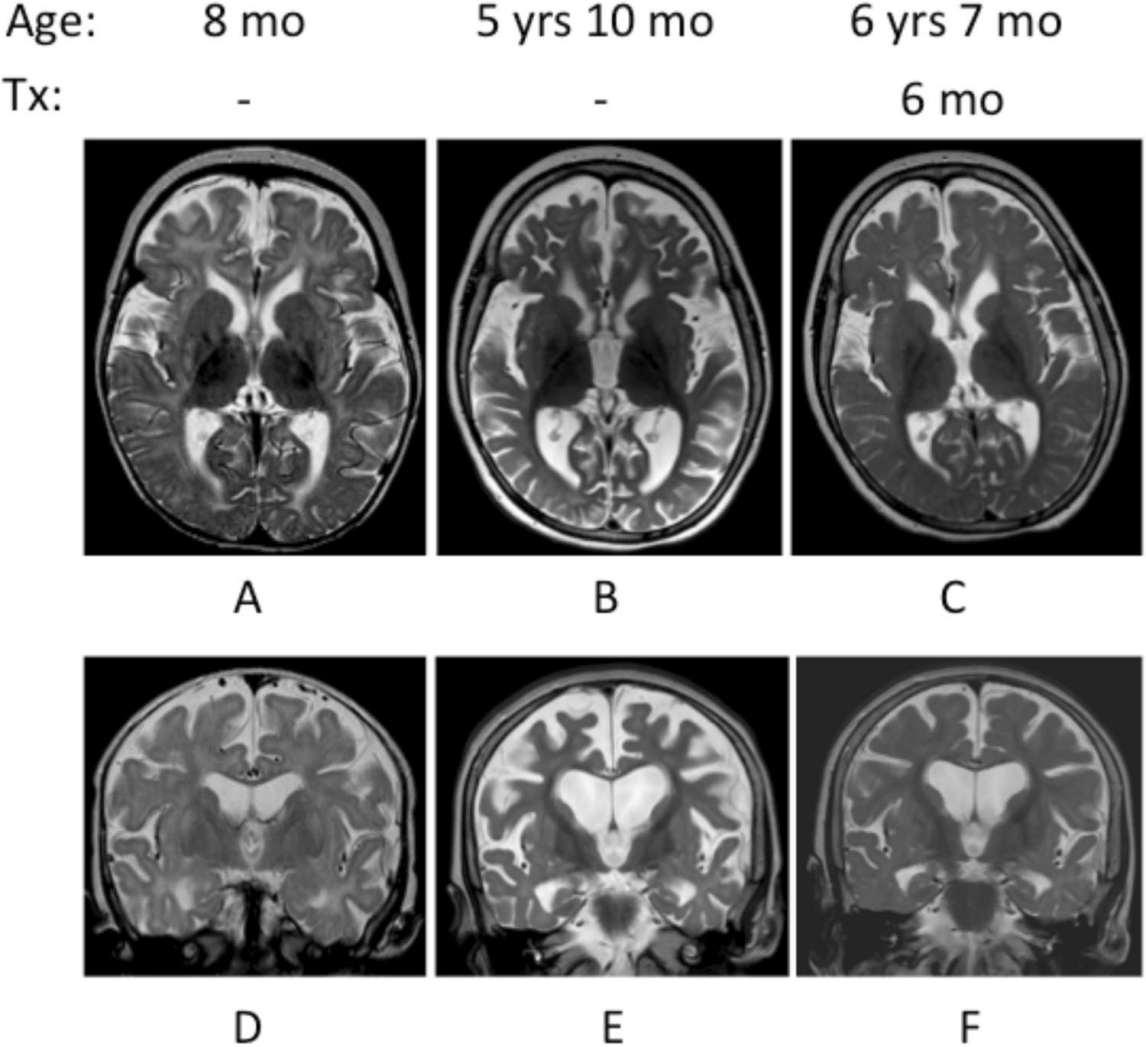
Results from MRI investigations in a patient with AGC1 deficiency before and 6 months after treatment [110]. Before treatment, T_2_ axial imaging A, B and T_2_ coronal imaging D, E showed lack of myelination and progressively reduced supratentorial cerebral volume. At 6 years and 7 months of age, after 6 months of treatment with the ketogenic diet, T_2_ axial imaging (C) and T_2_ coronal imaging F show that the previously high signal corresponding to white matter is lower, and that the ventricles and subarachnoid spaces are less prominent, indicating reversal of the volume loss. Tx, duration of treatment with the ketogenic diet. Reproduced from [110] with permission
